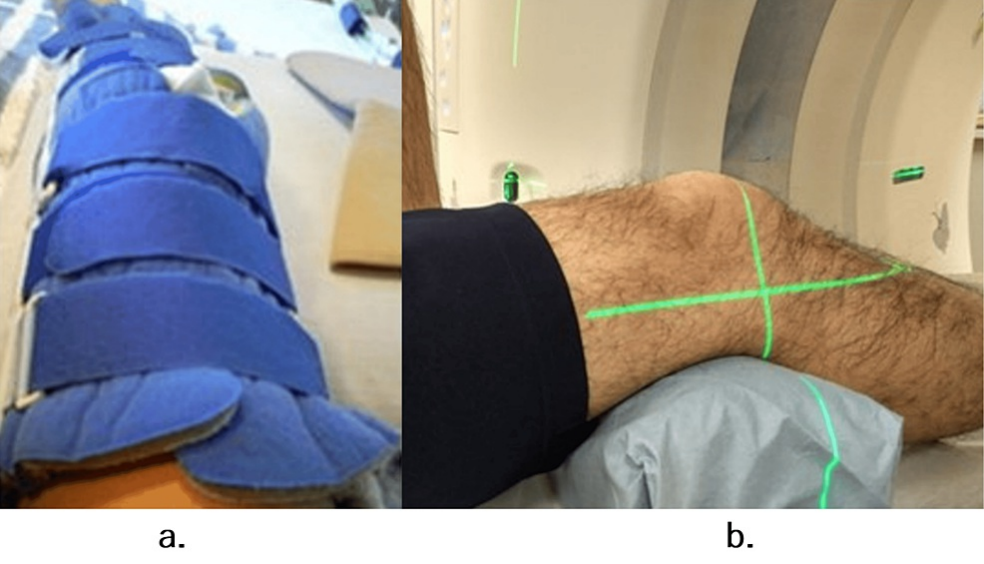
Postoperative CT scan imaging. Follow-up CT after ACL surgery is shown. Remove the knee brace for fixing the knee (a) and maintain the position below the knee joint using an auxiliary tool to maintain the same positioning as that for fixation (b).
